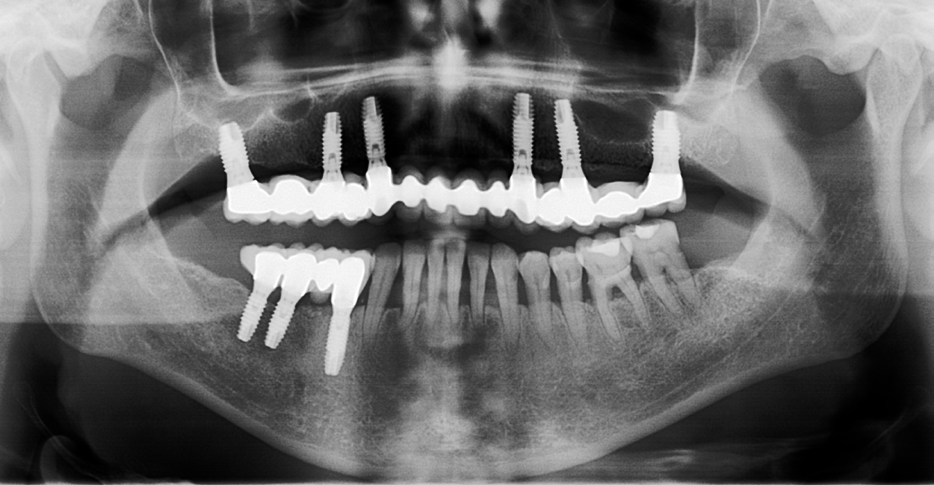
Panoramic radiography after final prostheses placement.
